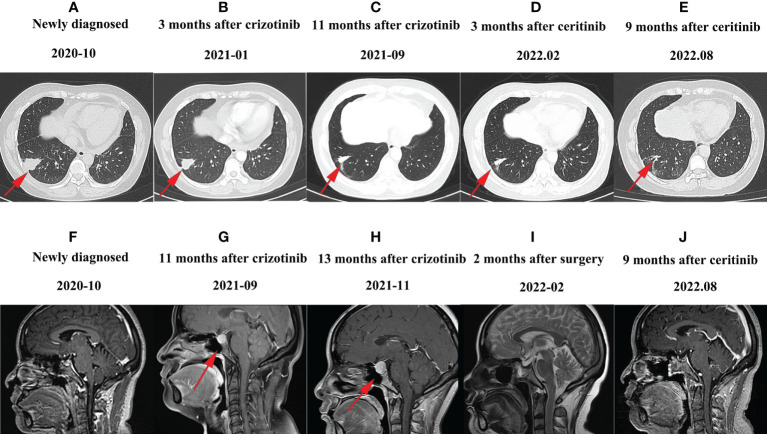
Representative CT or MRI images of the patient: (A) CT image of October 2020 before the treatment. (B) CT image of January 2021 after treatment with crizotinib. The therapeutic effect of the patients was evaluated as partial response. (C) CT image of September 2021 after pituitary metastases; the lung lesion was stable. (D) CT image of February 2022 after treatment with ceritinib; the lung lesions shrank further. (E) CT image of August 2022 after treatment with ceritinib; the lung lesion is still shrinking. (F) MRI image obtained in October 2020 before treatment; brain MRI showed no abnormalities. (G) MRI image of September 2021 after finding pituitary metastases. (H) MRI image after 1 month of supplemental hormone therapy. The pituitary gland was enlarged. (I) MRI image after sphenoid sinus exploration and nasal endoscopic saddle area tumor excision, and the brain MRI showed no mass. (J) Follow-up MRI images 9 months after tumor resection and 9 months after ceritinib administration.
